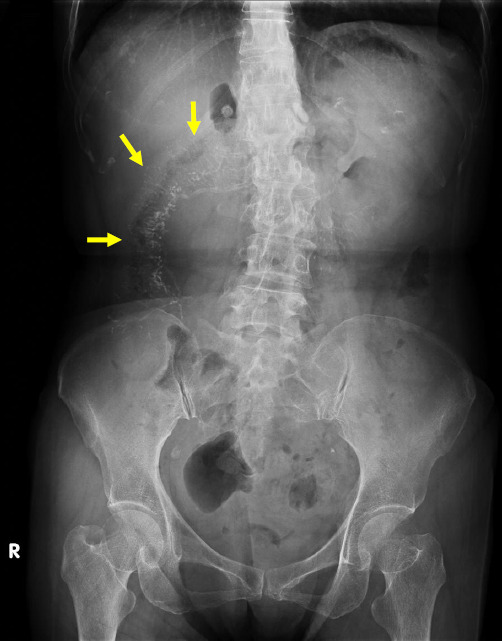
Abdominal plain film of the patient shows dendritic serpiginous radiopaque opacities alongside the ascending and transverse colon.
